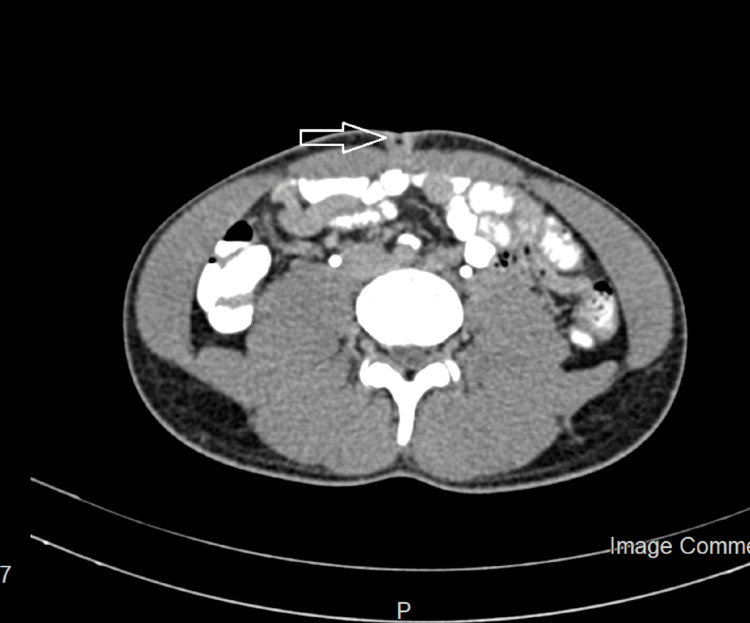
The CT Scan showing urachal sinus at the umbilicus
